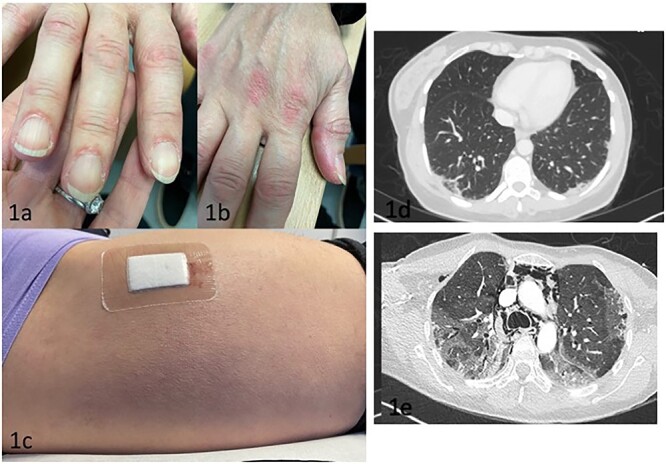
Images of the skin and CT changes seen in case A (a) Right hand digits showing periungual swelling and erythema (b) Gottron’s papules on the right second and third metacarpophalangeal joints. Rough and cracked skin can be seen on the lateral aspect on the thumb, consistent with mechanic’s hands. (c) Right lateral thigh showing a diffuse erythematous rash, in keeping with the Holster sign. (d) CT imaging on initial presentation showing mild left lower lobe subpleural consolidation and right lower lobe patchy consolidation (e) Interval CT imaging 6 weeks later showing rapidly progressive ground-glass changes and pneumomediastinum.
